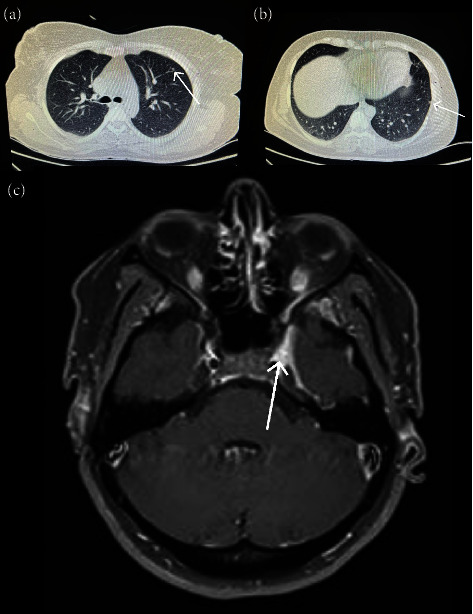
(a, b) Computed tomography scan of lung demonstrating lung nodule; (c) magnetic resonance imaging demonstrating soft tissue enhancement in the left cavernous sinus and superior orbital fissure, with no pressure effect on the carotid artery, in favor of Tolosa–Hunt syndrome.
